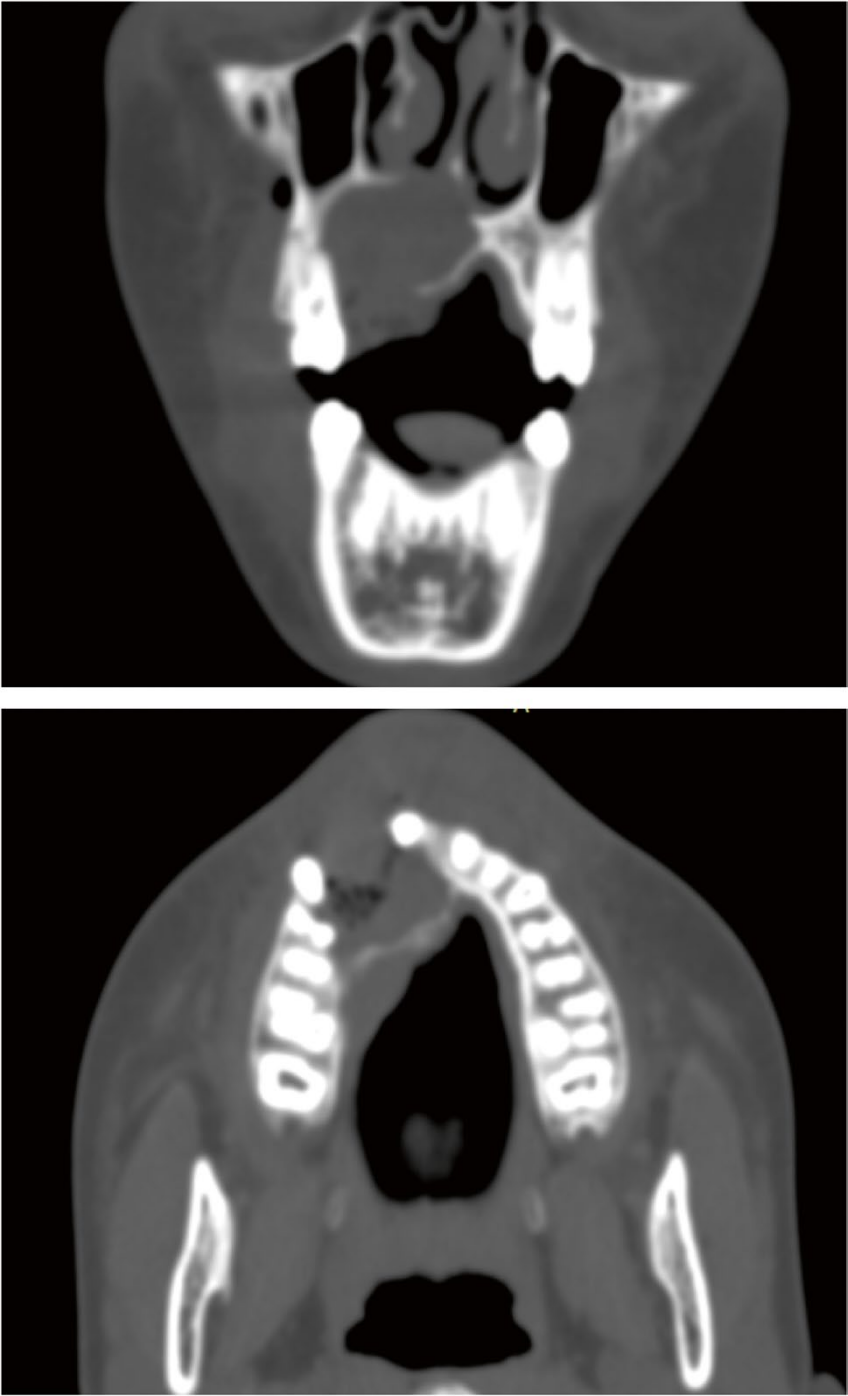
CT scan coronal and axial cuts showing bony defect after removal of cystic lesion and palatal thickening
